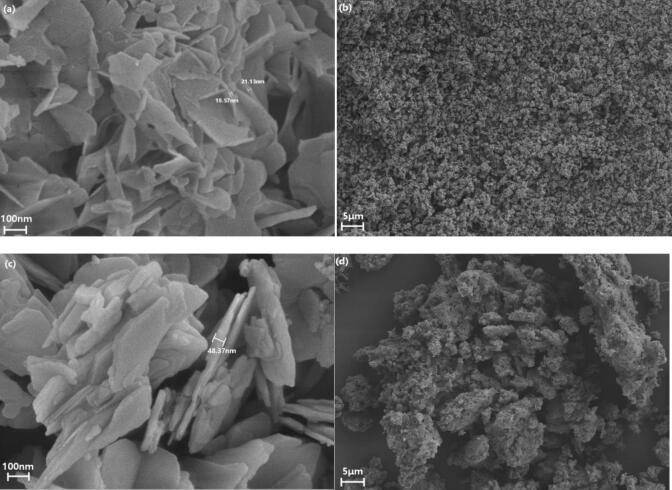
SEM comparison of products prepared under ultrasonic–jet and conventional jet conditions.
